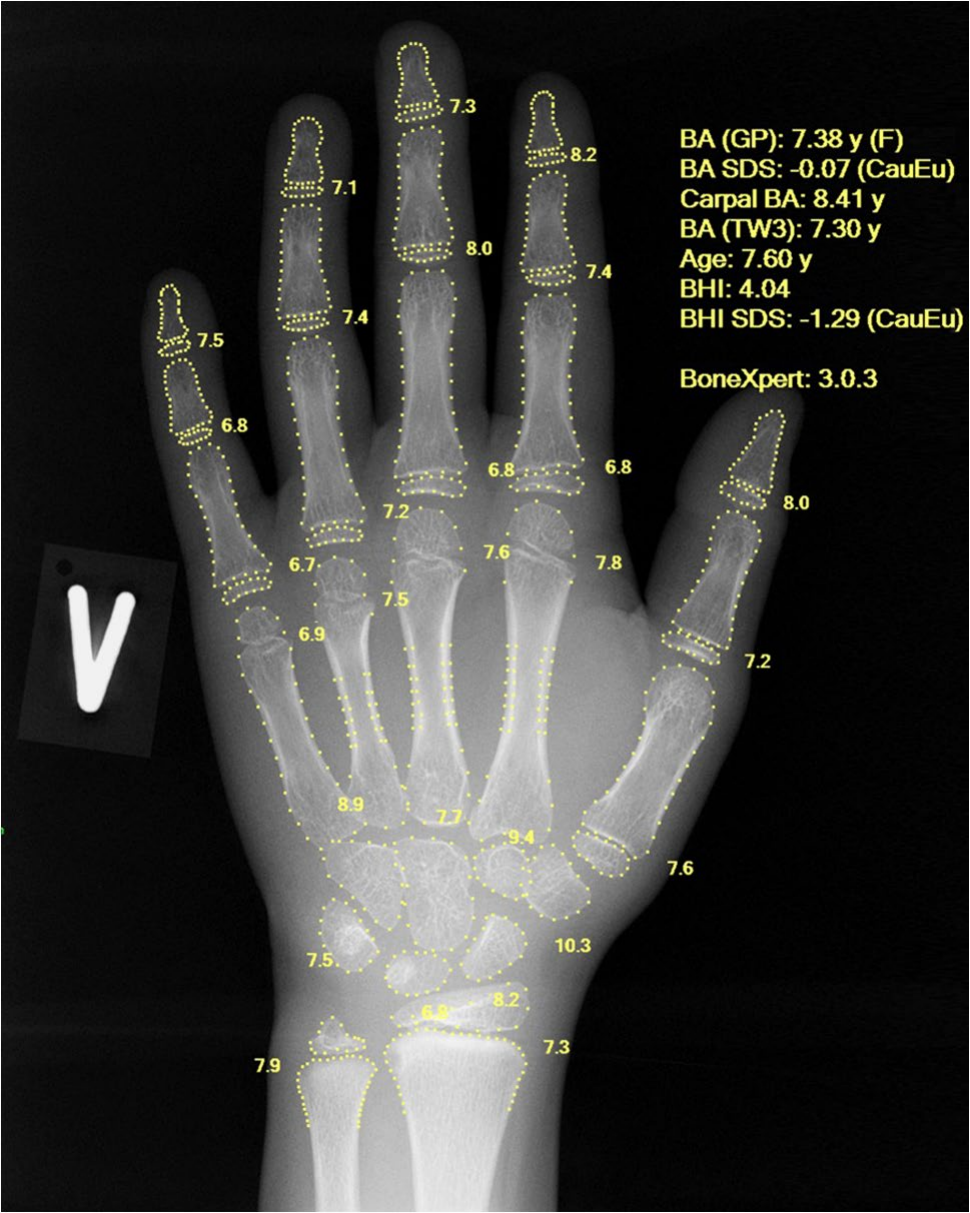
Dorsopalmar left hand radiograph in a 7.6-year-old girl following bone age assessment by BoneXpert. The output of the artificial intelligence (AI) system is an annotated Digital Imaging and Communications in Medicine (DICOM) file placed in the same study in the hospital’s picture archiving and communications system (PACS) as the original image. The algorithm has located the borders of the bones and assigned a Greulich and Pyle (GP) bone age to each of them. The average bone age (BA) for the 21 tubular bones is reported as “BA (GP): 7.38 y (F),” where the F indicates female gender, as taken from the DICOM header. The next line reports a bone age standard deviation score (SDS) of –0.07, which means that the bone age is 0.07 standard deviations below what is expected at that chronological age. Chronological age is indicated below the bone age SDS as 7.60 years (computed from the birth and study dates in the DICOM header). The remaining reported numbers are: carpal BA = the average bone age in the seven carpals, BA (TW3) = Tanner and Whitehouse version 3 bone age, BHI = bone health index, and its SDS relative to girls with the same bone age
